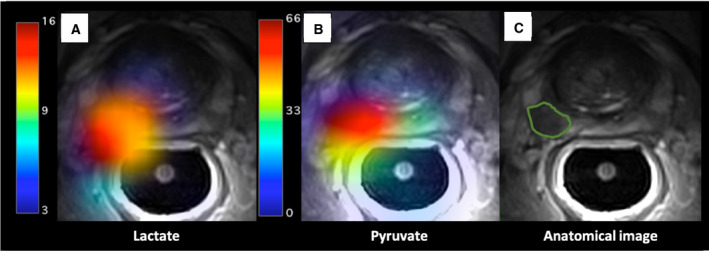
Hyperpolarised 1‐^13^C‐pyruvate‐MRI metabolite maps in MRI‐ and biopsy‐positive PCa. Axial T2‐weighted ^1^H‐MRI scan of a subject with right‐sided, posterior, biopsy‐confirmed Gleason 4 + 3 PCa, segmented in a green line in panel C. Images were acquired with a receive‐only endorectal coil (RAPID Biomedical). Maps of 1‐^13^C‐pyruvate (centre, panel B) and 1‐^13^C‐lactate (left, panel A) were produced, via an IDEAL model, from multiecho‐bSSFP data (13C‐MRI) and overlaid on the original T2W acquisition; demonstrating the distribution of hyperpolarised 1‐^13^C‐pyruvate (panel B) and its downstream metabolites, (lactate in panel A) 25 s after contrast injection.
